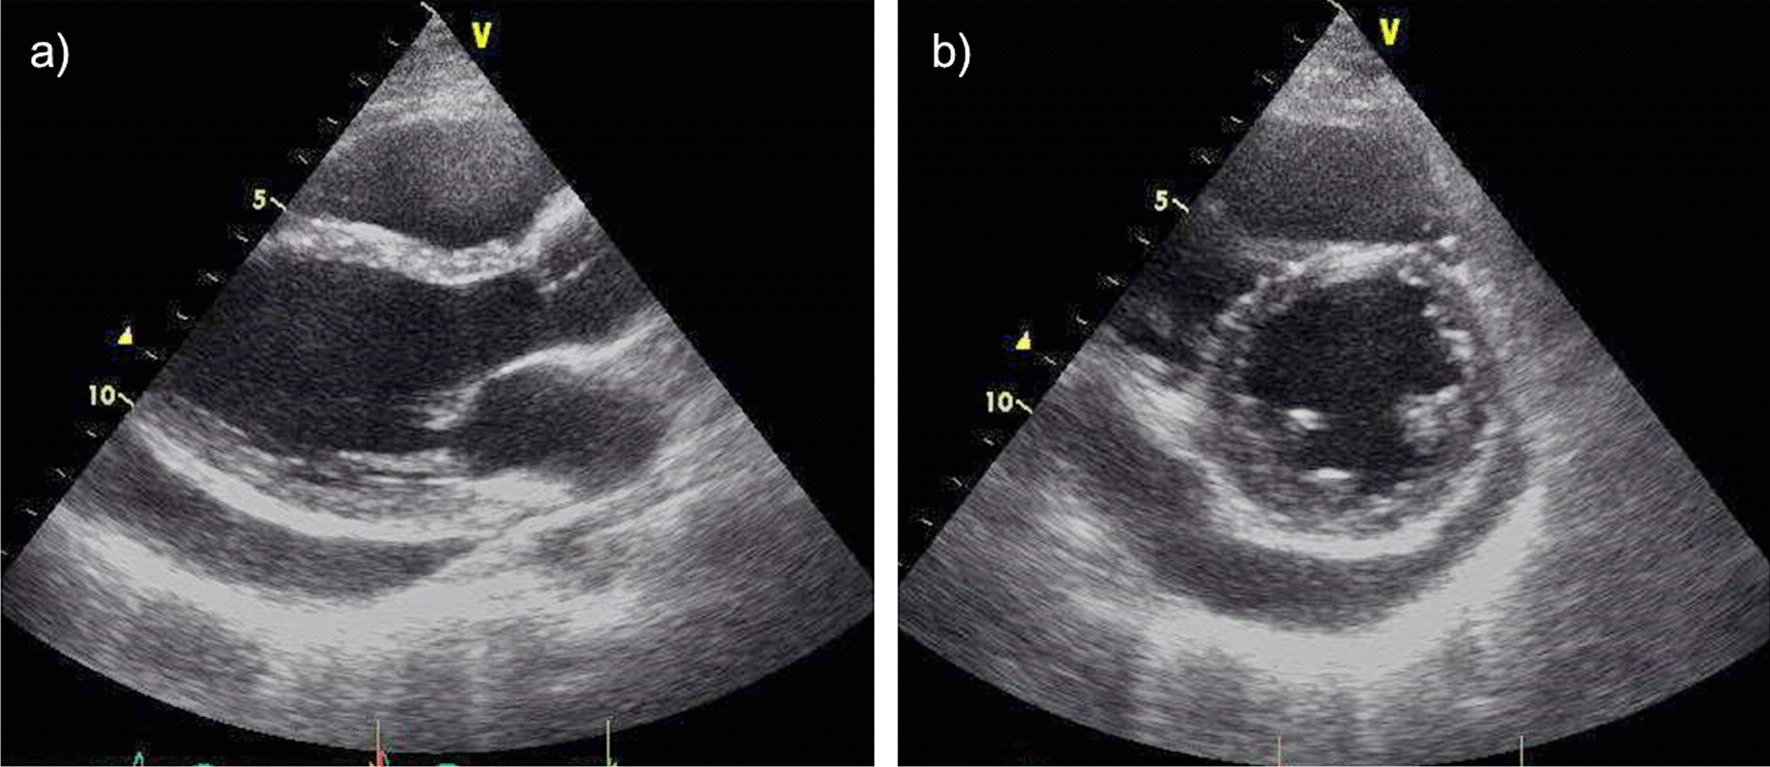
Transthoracic echocardiogram at the initial visit. a Parasternal left border long-axis tomogram, b parasternal left border short-axis tomogram. Echocardiography showing pericardial effusion
